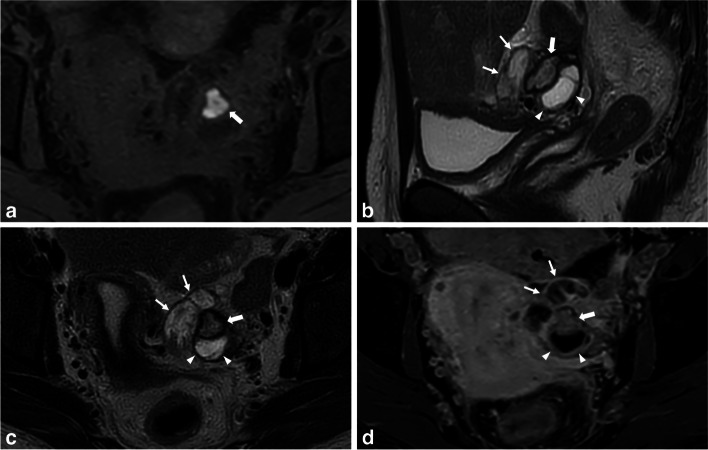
Subacute tubo-ovarian abscess in a 30-year-old woman with subacute pelvic pain (3 weeks ago) and persistent left pelvic pain. a Axial T1-W fat-suppressed MR image shows a T1-hyperintense cystic ovarian lesion (thick arrow), corresponding to proteic and hemorrhagic content. b Sagittal and (c) axial T2-W MR images show an ovarian well-defined cystic cavity with intermediate T2-signal intensity, surrounded by a hypointense peripheral rim (thick arrows). Note the presence of an adjacent hydrosalpinx with heterogeneous signal intensity (thin arrows). Of note, the presence of a homolateral T2-hyperintense functional ovarian cyst (arrowheads). d Axial T1-W fat-suppressed contrast-enhanced MR image shows enhancement of the collapsed walls of the remnant ovarian abscess (thick arrow) as well as the walls of the hydrosalpinx (thin arrows), with obliteration of the fat planes around the left ovary. Of note, the presence of a homolateral functional ovarian cyst showing a peripheral enhancement (arrowheads)
